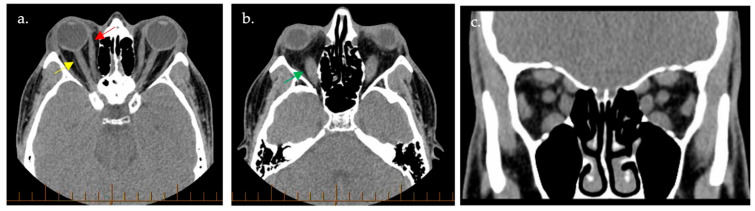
Computed tomography images demonstrated extraocular muscle enlargement in thyroid eye disease. Computed tomography (CT)-based images in axial (a,b) and coronal (c) planes showcase superior (yellow arrow), medial (red arrow), and inferior (green arrow) rectus muscle enlargement bilaterally.
